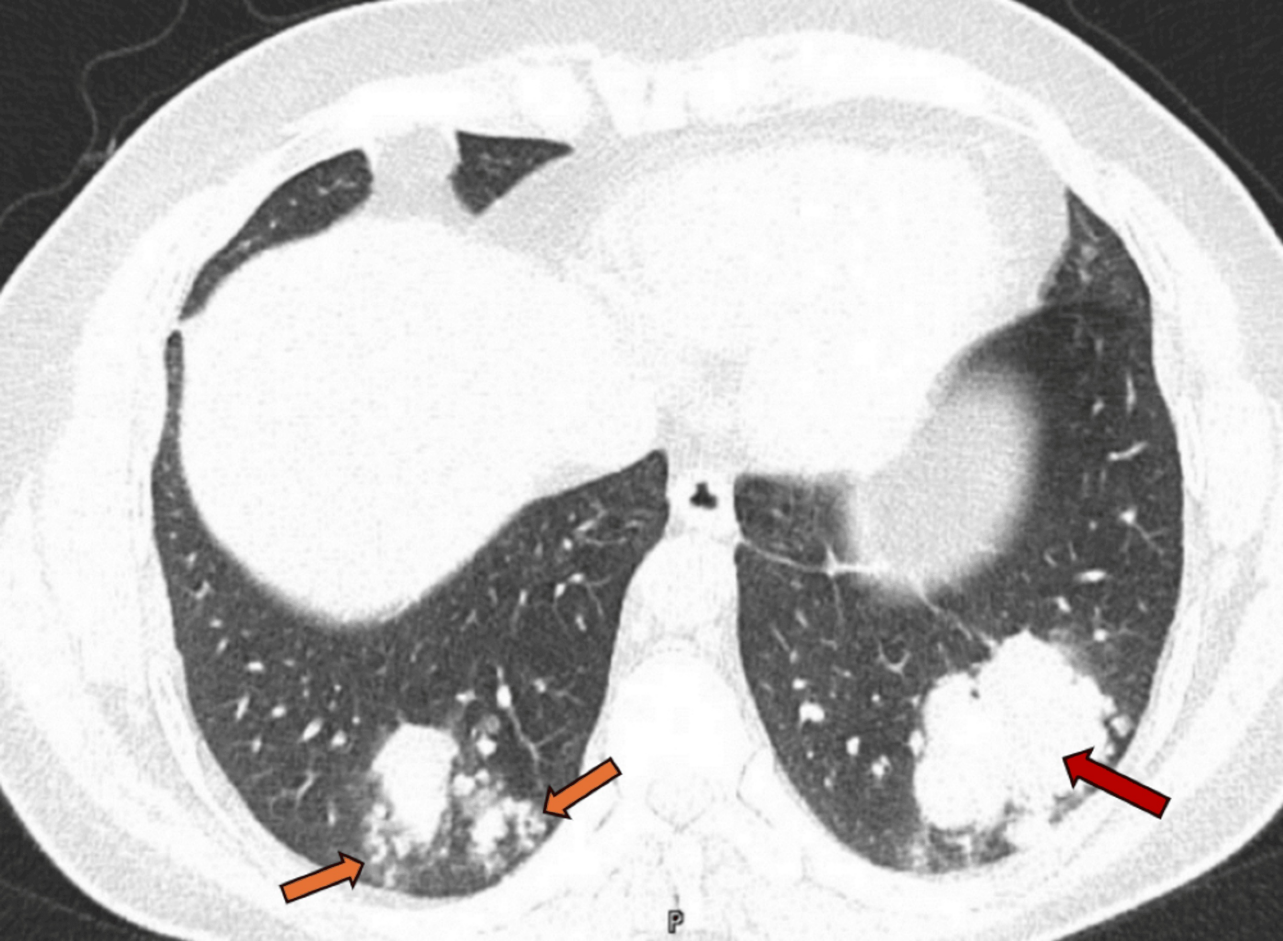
A CT scan of the chest (axial image in the lung window) shows bilateral basal mass-like lobulated opacities. Small nodular opacities are also seen surrounding the lesions (orange arrows). A biopsy was obtained from the large lesion in the left lower lobe (red arrow).
